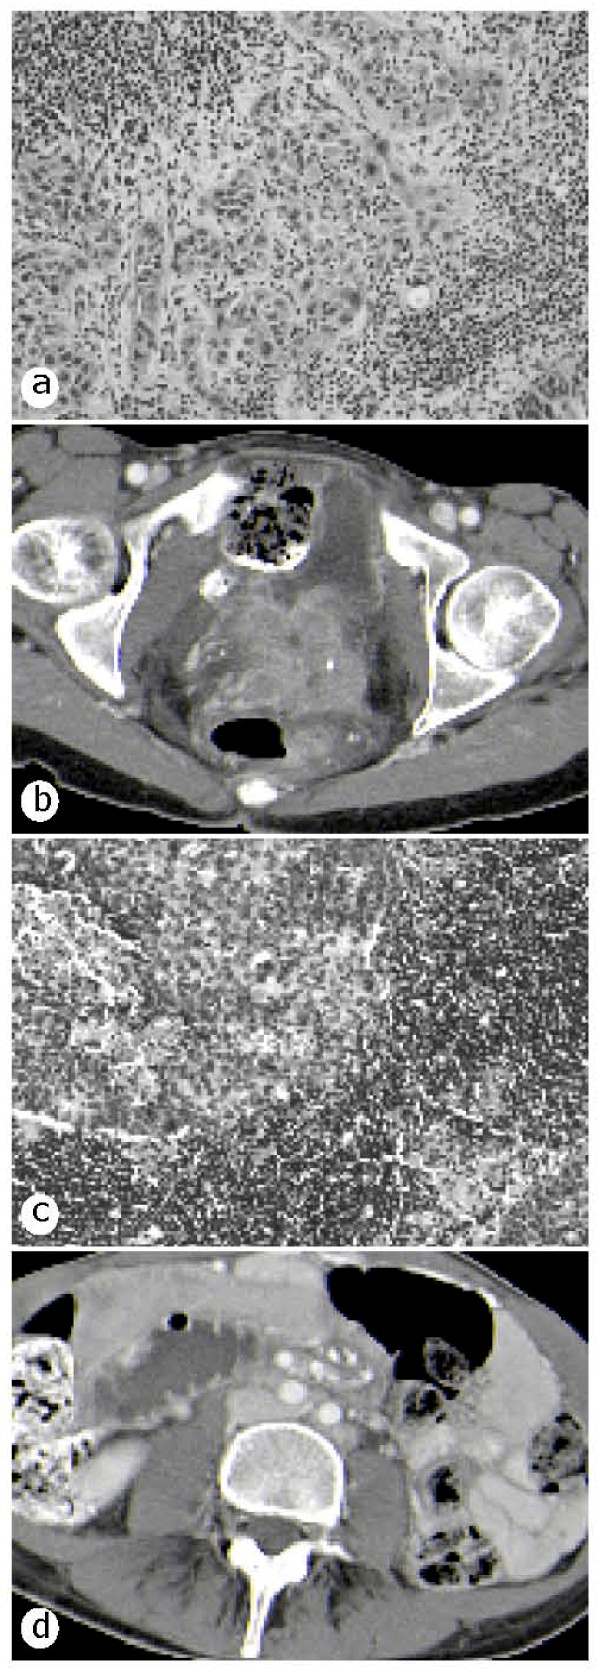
Restaging of the tumor. (a) formalin-fixed, paraffin-embedded biopsy of the invasive cervix cancer; (b) corresponding CT scan of the pelvis; (c) formalin-fixed, paraffin-embedded biopsy of the para-aortic lymph node metastases and her abdomen showing bladder invasion; and (d) corresponding CT scan of the enlarged para-aortic lymph nodes.
